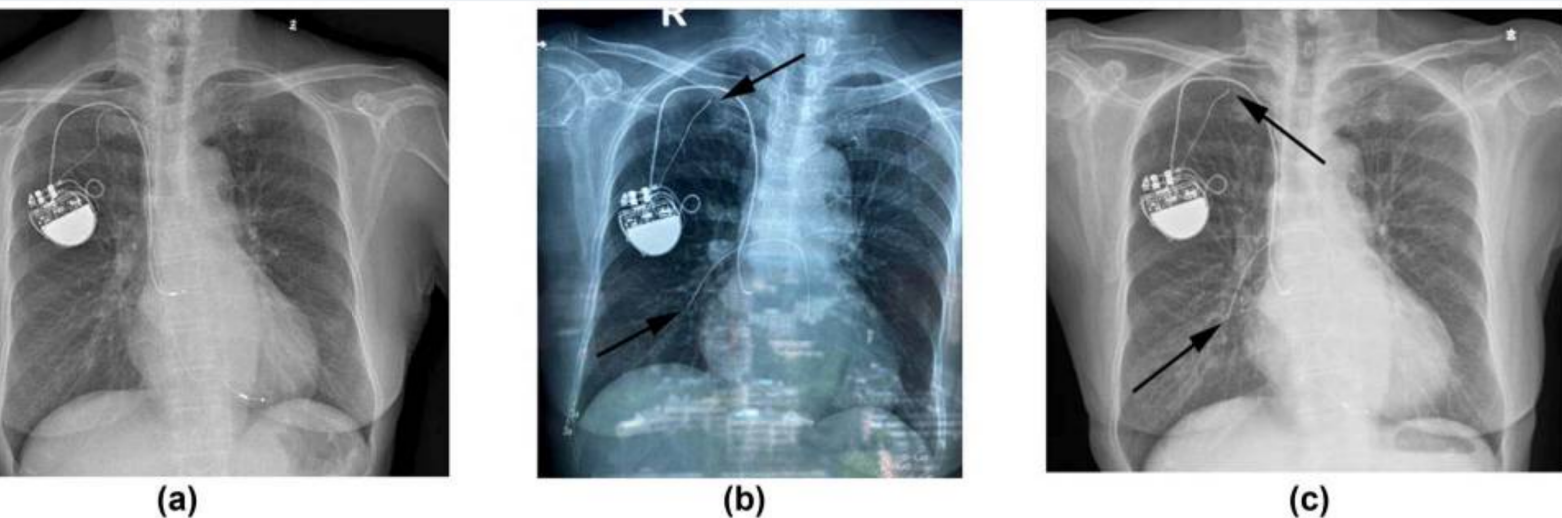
Chest X-ray images (a) showing both leads were intact, while (b)
